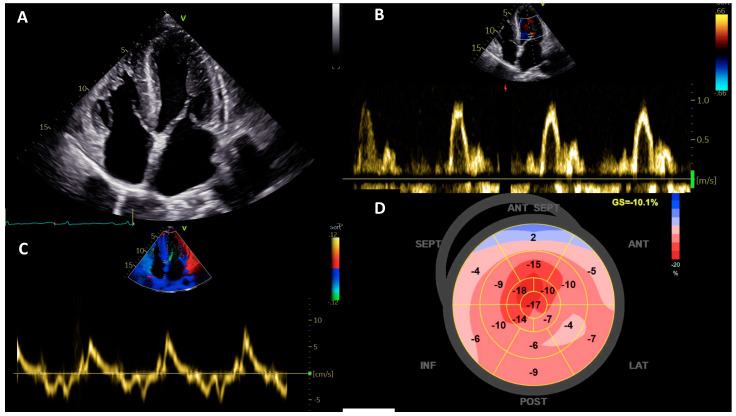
Echocardiographic clues of a patient with AL cardiac amyloidosis (A): Apical four-chamber view demonstrates increased biventricular wall thickness with a sparkling texture of the myocardium, thickening of the interatrial septum, and biatrial enlargement. (B): Restrictive diastolic pattern. (C): Tissue Doppler imaging taken at the septal mitral annulus showing low e’ velocities. (D): Global longitudinal strain with the apical sparing pattern.
